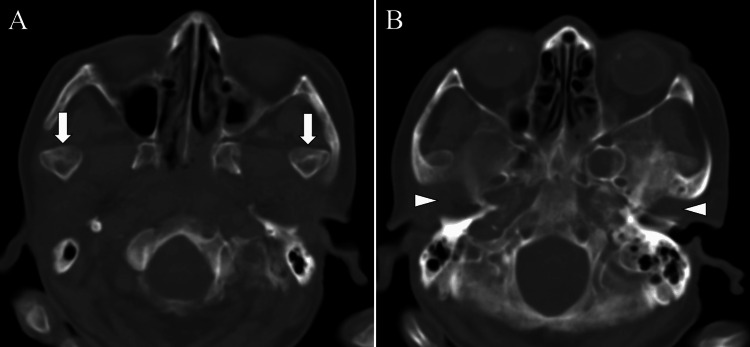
Head CT eight weeks after onset: CT revealing empty glenoid fossae in both temporomandibular joints (A; arrowheads) with anteriorly displaced condyles (B; arrows).
